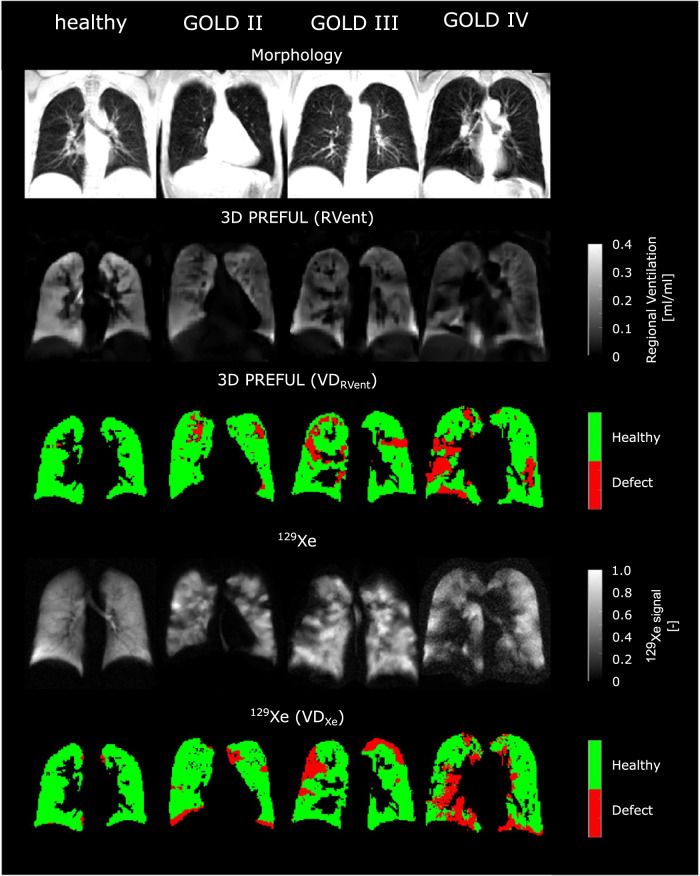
Representative morphological images (1st row) and ventilation parameter maps of four study participants derived by 3D PREFUL MRI (2nd and 3rd row, static RVent-based parameter maps) and ^129^Xe MRI (4th and 5th row). First column: a male 29-year-old healthy volunteer (FEV_1_ = 80% pred., FVC = 85% pred.); second column: a female 67-year-old COPD patient (FEV_1_ = 66% pred., FVC = 103% pred., GOLD II); third column: a male 60-year-old COPD patient (FEV_1_ = 39% pred., FVC = 66% pred., GOLD III); fourth column: a male 70-year-old COPD patient (FEV_1_ = 28% pred., FVC = 64% pred., GOLD IV)
